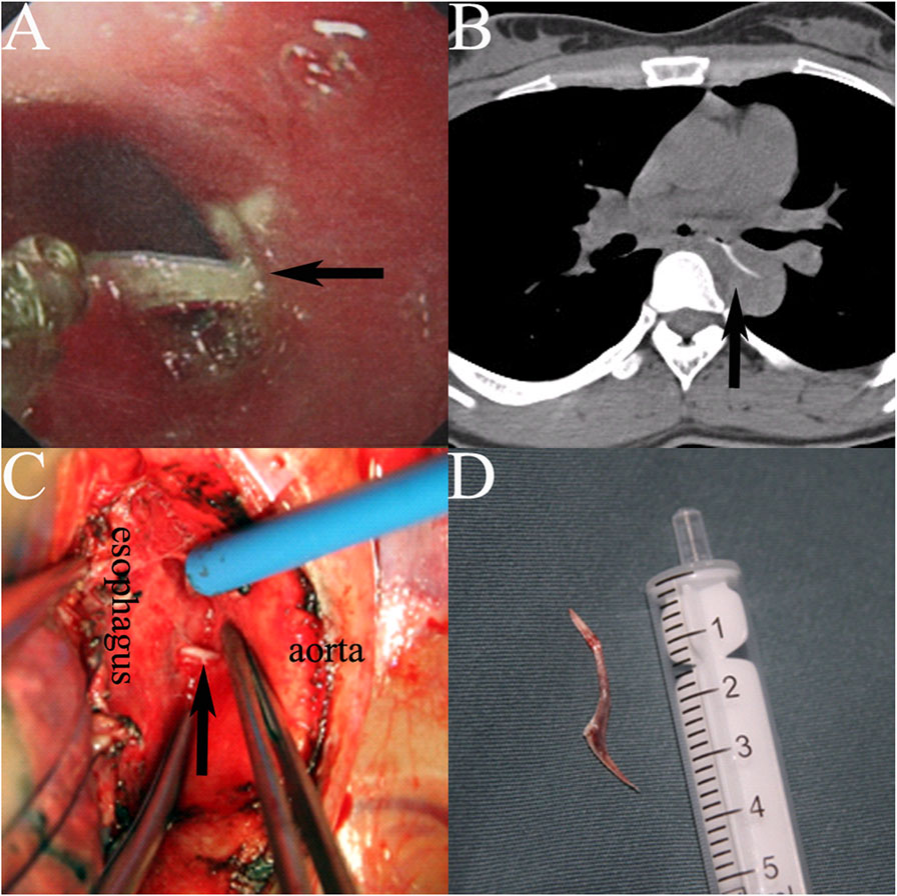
a A typical picture of gastroscopy demonstrats the fish bone (arrow) piercing into the esophagus wall; b A CT shows a fish bone (arrow) in esophagus piercing directly into the thoracic aorta. c Fish bone (arrow) piercing from esophagus into the aorta (Intraoperative vision). d Fish bone after removal
